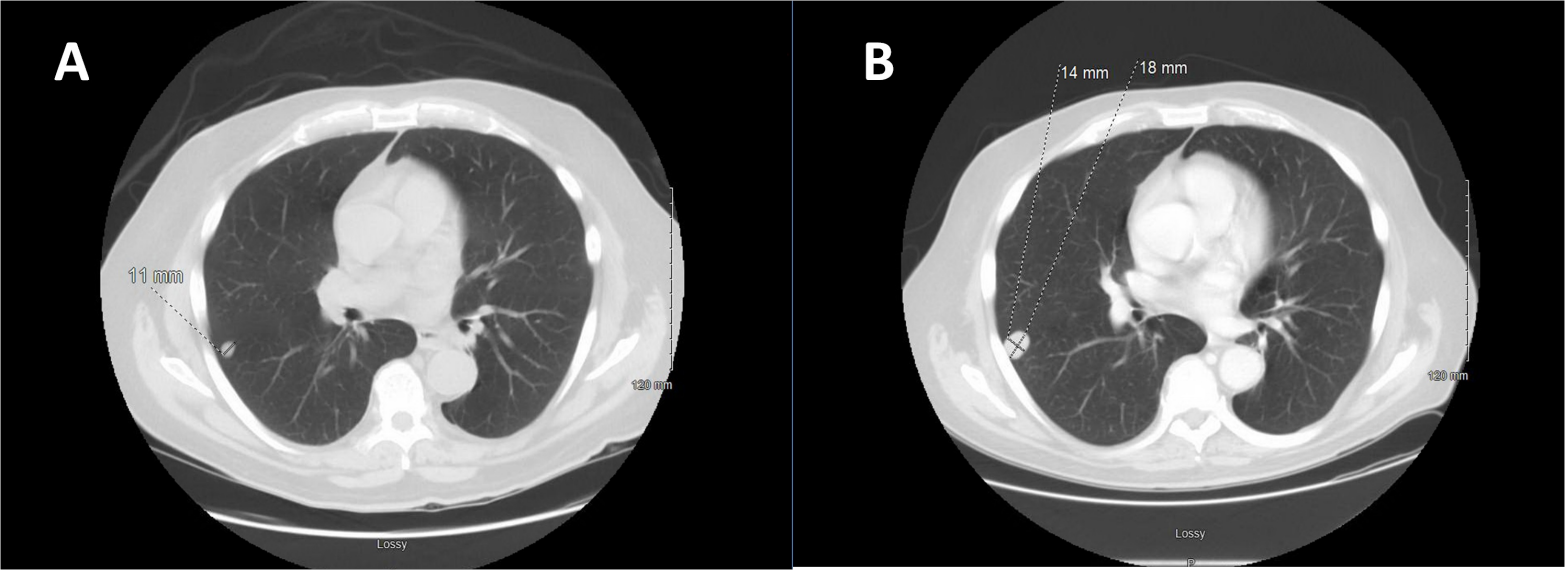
CT Chest at Diagnosis and After One Cycle of Combination Chemotherapy A: CT scan of the chest at diagnosis reveals a 1.1 cm nodule in the right lower lobe. B: CT scan of the chest after one cycle of gemcitabine/docetaxel reveals an increase in the size of the right lower lobe pulmonary nodule (1.4 x 1.8 cm).
